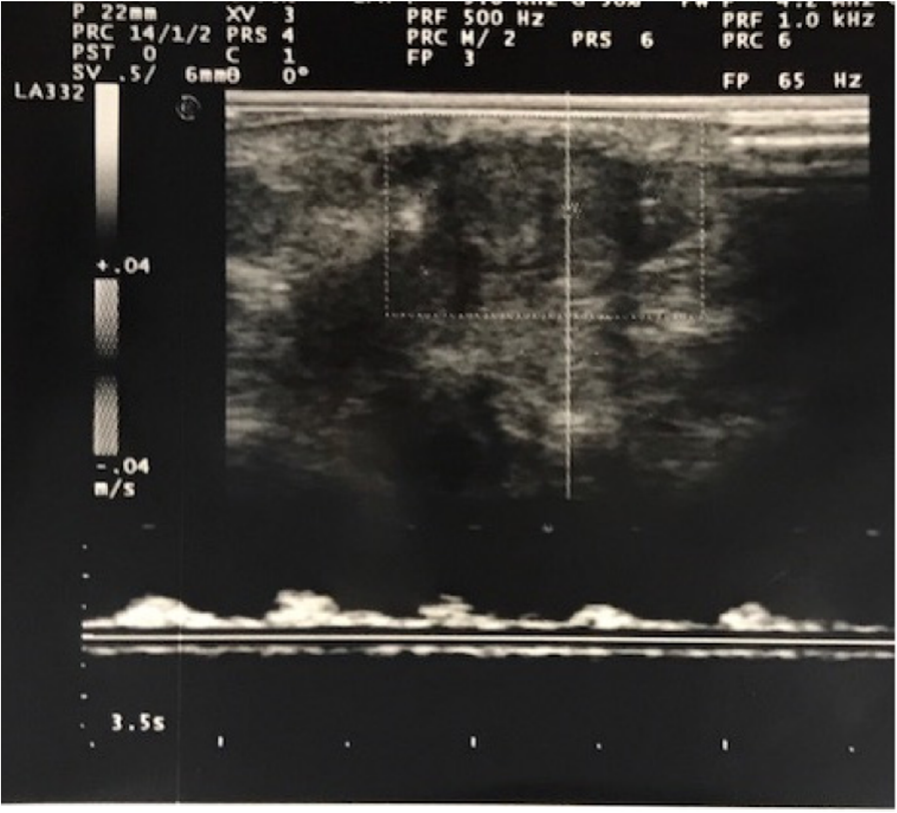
Color Doppler ultraonography *Normal arterial flow on Colour Doppler Ultrasonography (CDU) of the penis in our newborn with priapism*
